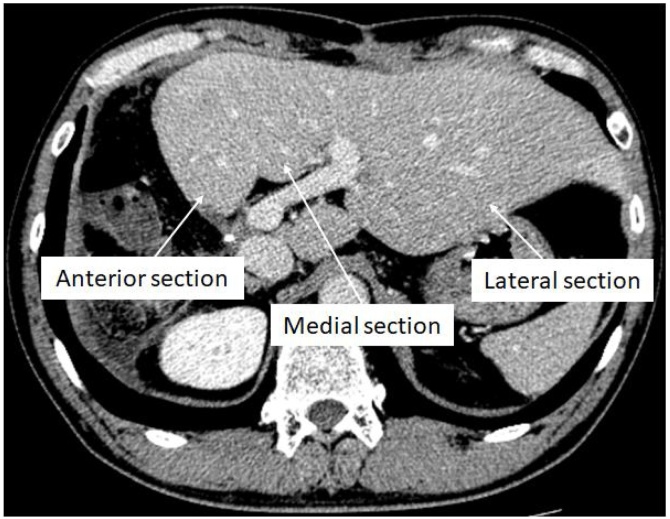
The remnant liver remains in the anatomical position, and medial and anterior sections are well enhanced on computed tomography.
